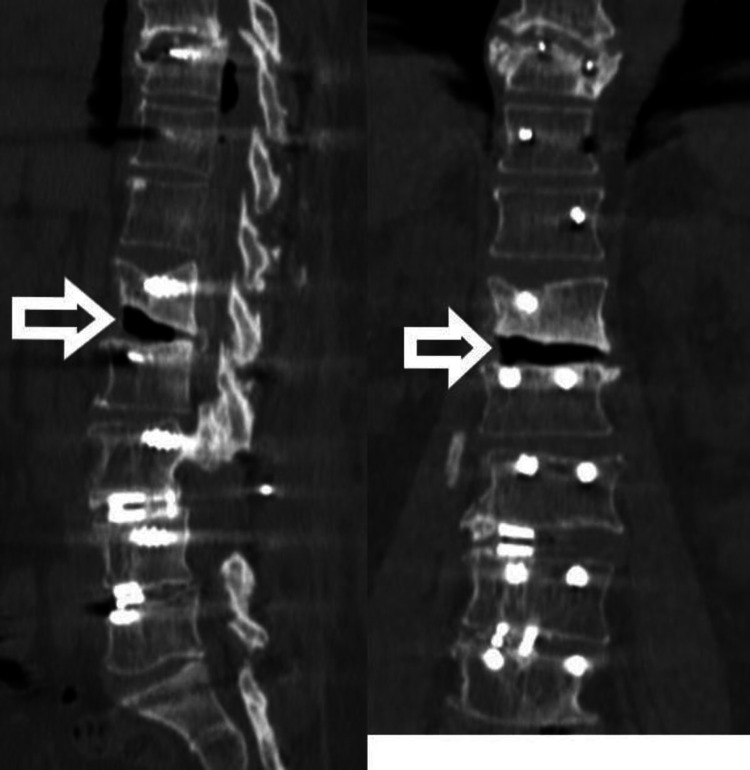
CT scan showing “vacuum sign” at the site of rod breakage
